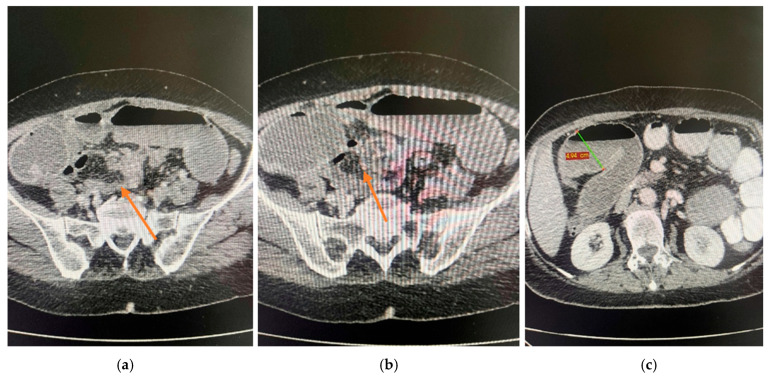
CT examination of the ileum. (a) Focal irregular parietal thickening (orange arrow). (b) Stenosing lesion (orange arrow). (c) Upstream intestinal dilatation (green measurement line).
